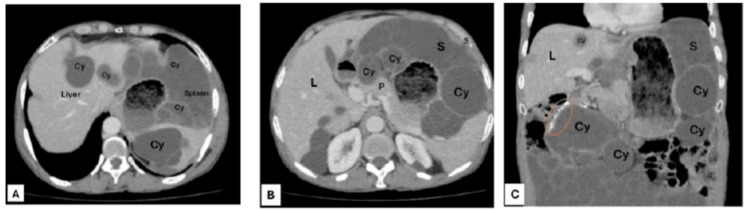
A. CT scan of the abdomen (axial section) showing hydated cysts (Cy) in the liver (L) and spleen (S). (B) Multiple multilocular fluid density lesions (Cy) anterior to the pancreas (P) and spleen (S), demonstrating a spoke wheel appearance characteristic of hydatid cysts (Cy). (C) A large, well-defined multivesicular cyst (Cy) inferior to the liver (L) showing thick peripheral wall calcification (orange circle). Other cysts (Cy) are also present inferior to the spleen (S).
